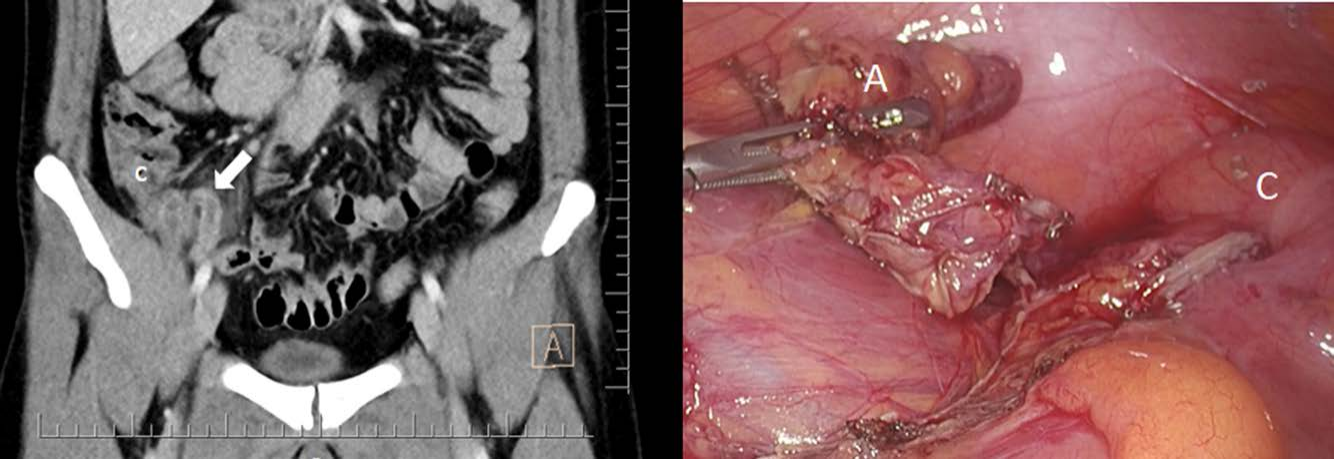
Computed tomography scans performed at the external center during the first emergency service admission of the patient, and intraoperative scene. The blind-ended and thickened-walled intestinal structure (white arrow). C = caecum, A = appendix.
